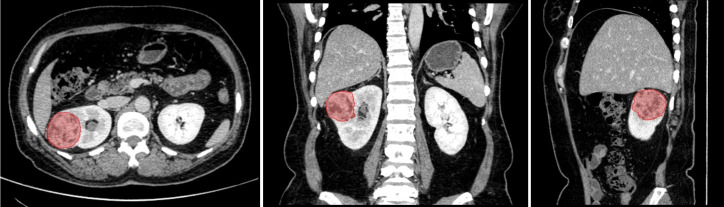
The segmentation of entire tumor mass (red region) on nephrographic phase CT. From left to right are axial, coronal and sagittal.
